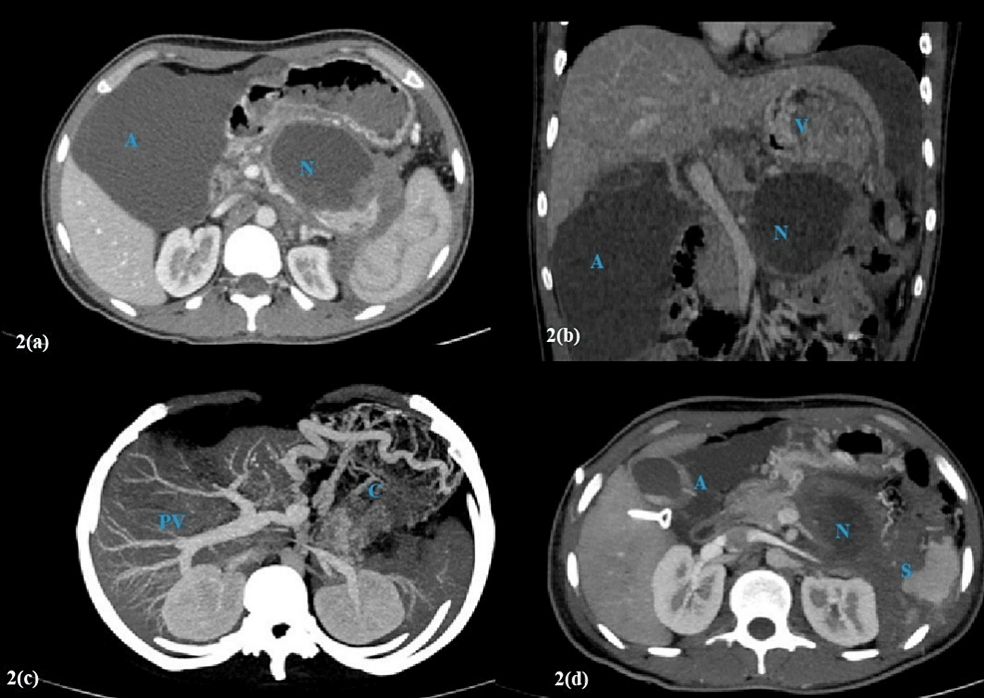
Pre- and post-embolization CECT findings. (a) and (b) are axial and coronal reformatted images showing ascites (A), pancreatic necrosis (N), and varices (V). The portal vein appears normal. (c) is an axial maximum intensity projection image before the procedure showing normal opacification of the portal vein (PV) and its branches and perigastric collaterals (C). Splenic is not visualized. (d) is an axial CECT image, post-partial splenic embolization which shows a partially infarcted spleen (S). Note the reduced lesser sac necrotic collection (N) and ascites (A). CECT: contrast-enhanced computed tomography.
